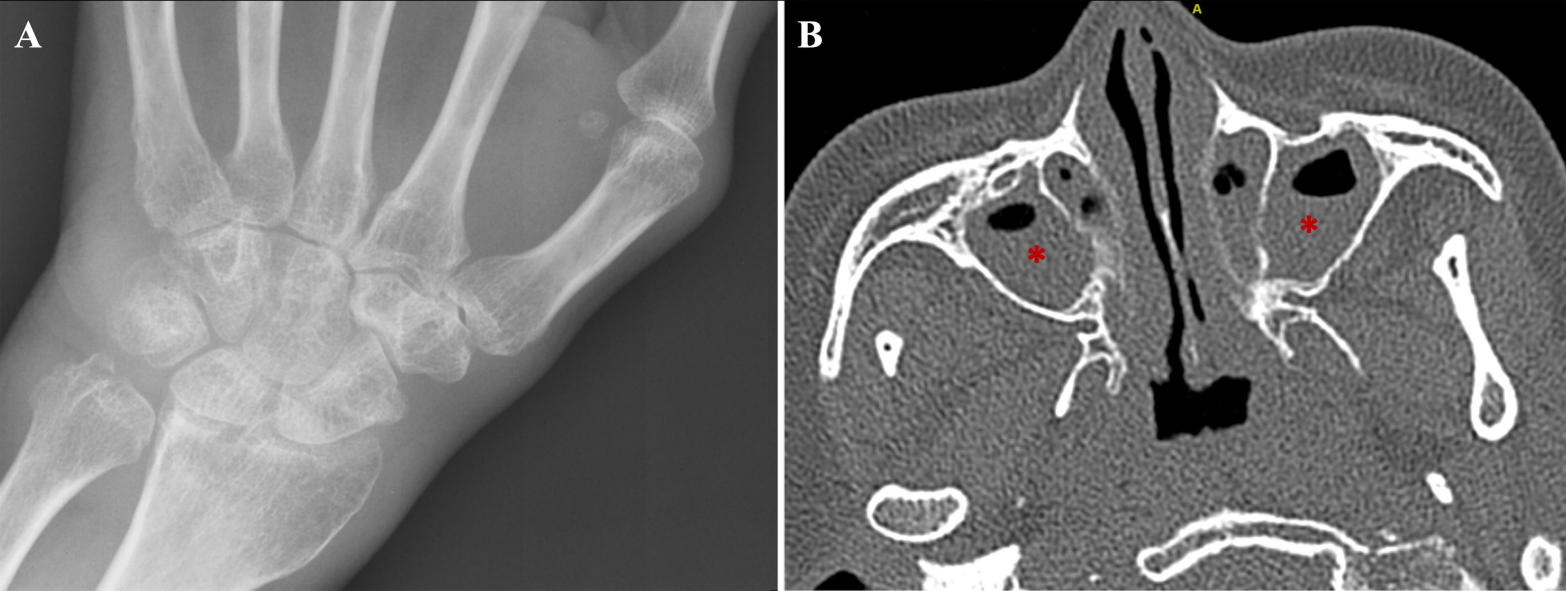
Representative imaging findings of RA and eosinophilic ENT involvement (HASM) in the patient. (A) Plain radiograph of the right wrist demonstrating characteristic erosive joint changes with periarticular osteopenia, consistent with longstanding rheumatoid arthritis (RA). (B) Axial computed tomography (CT) scan of the paranasal sinuses showing significant mucosal thickening and opacification (marked with red asterisks) in the maxillary sinuses bilaterally.
